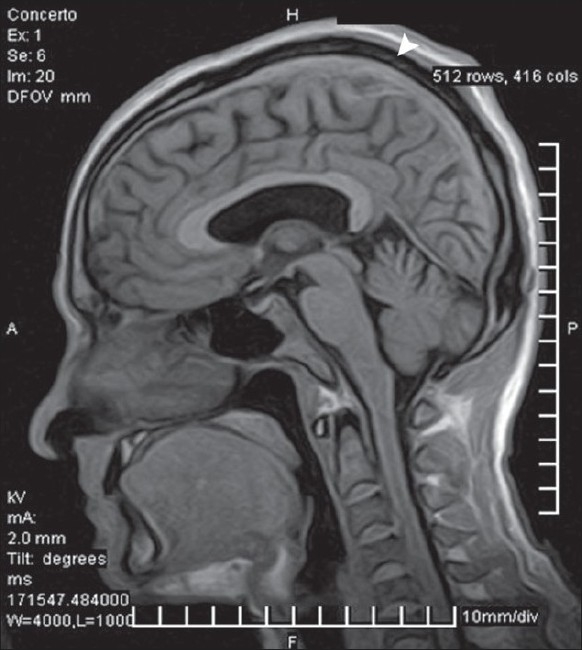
T1-weighted saggital magnetic resonance imaging showing hypointense lesion along the superior saggital sinus with abrupt discontinuity of the smooth contour, diagnostic of venous sinus thrombosis
